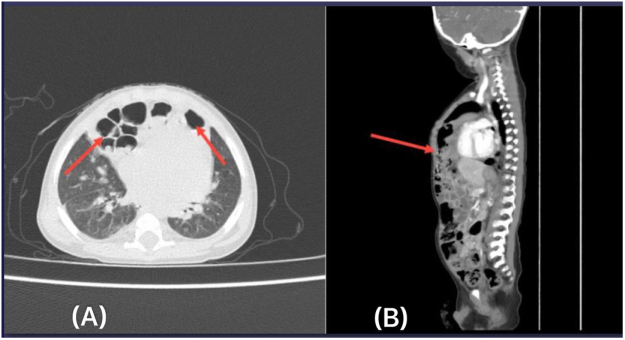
Chest CT scan (A: axial, B: sagittal) showing large bilateral anterior diaphragmatic hernia (with defect about 3 cm), located anterior to the heart, containing segments of the transverse colon, compatible with MH (red arrows), the hernia causing minimal compression on the left ventricle.
